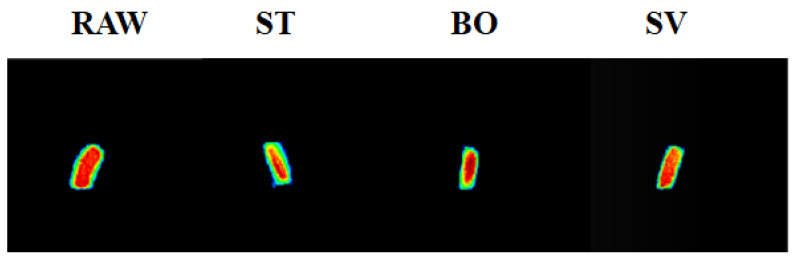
MRI images of the squid (*Symplectoteuthis oualaniensis*) mantle muscle with different cooking treatment. RAW: raw squid; ST: steaming; BO: boiling; SV: sous vide. The blue color indicates a lower proton density, while red color indicates a higher proton density.
